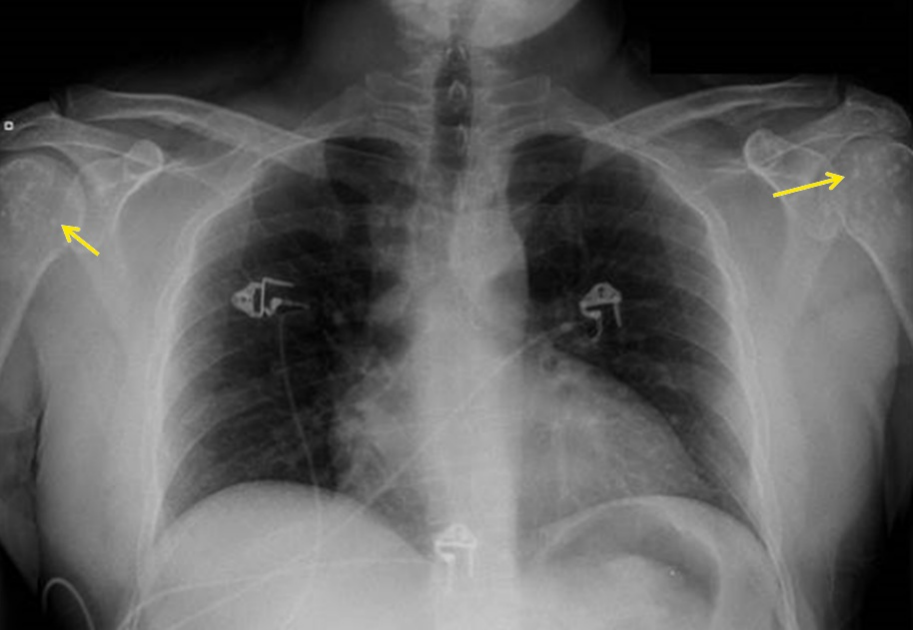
Posteroanterior Radiograph of the Chest. Non-aggressive periarticular sclerotic lesions (yellow arrows) are visualized at the bilateral glenohumeral joints.
